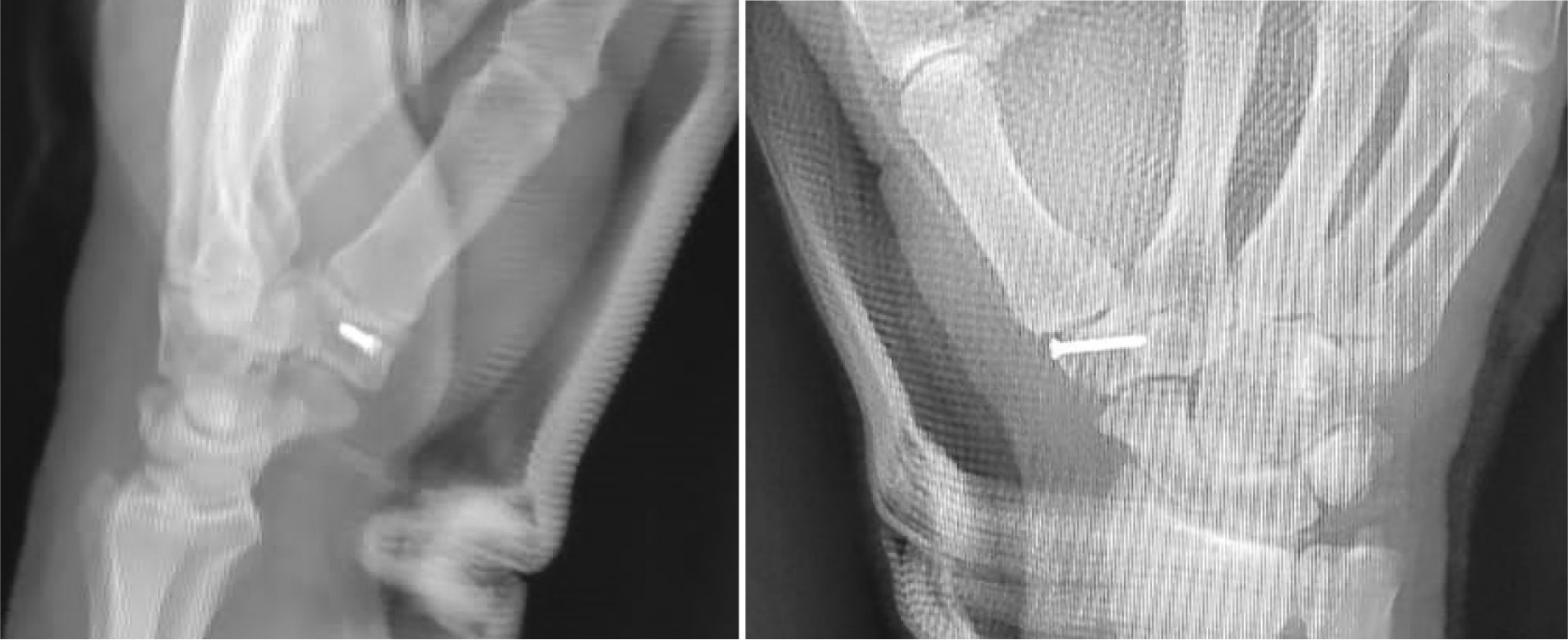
Standard postoperative control radiographs showing good compression of the fracture with the mini screw and the restoration of the articular surfaces.
